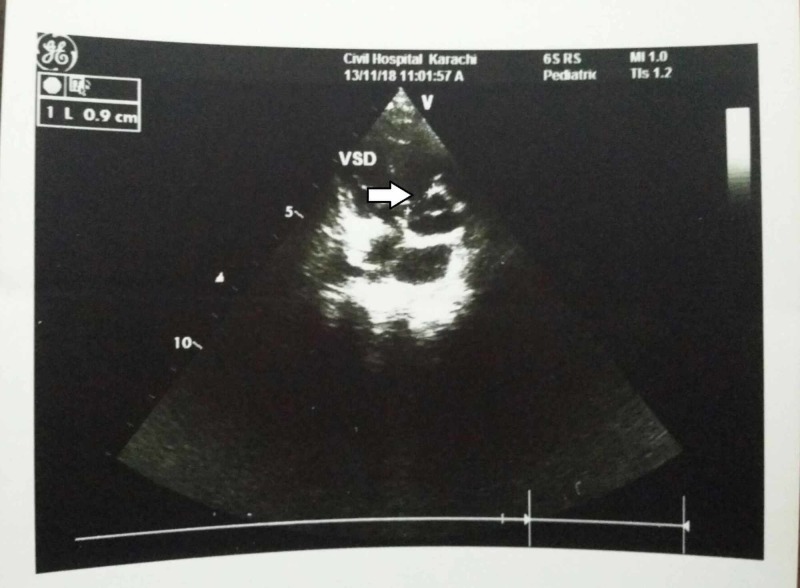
Transthoracic echocardiogram showing the ventricular septal defect
